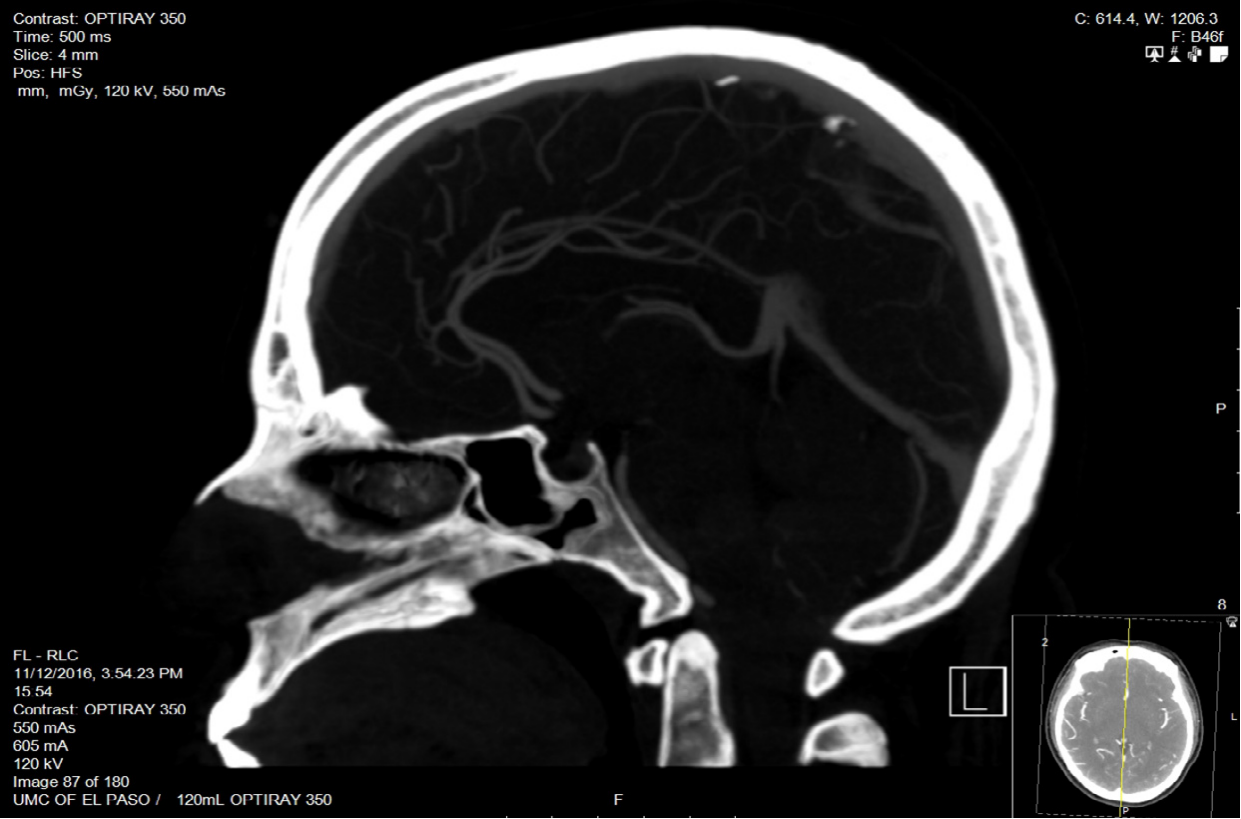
Computed tomography (CT) angiogram with IV contrast was negative for acute intracranial abnormalities, intra‐cranial arterial flow limiting stenosis, aneurysms, dissections, occlusion, or vascular malformations.
